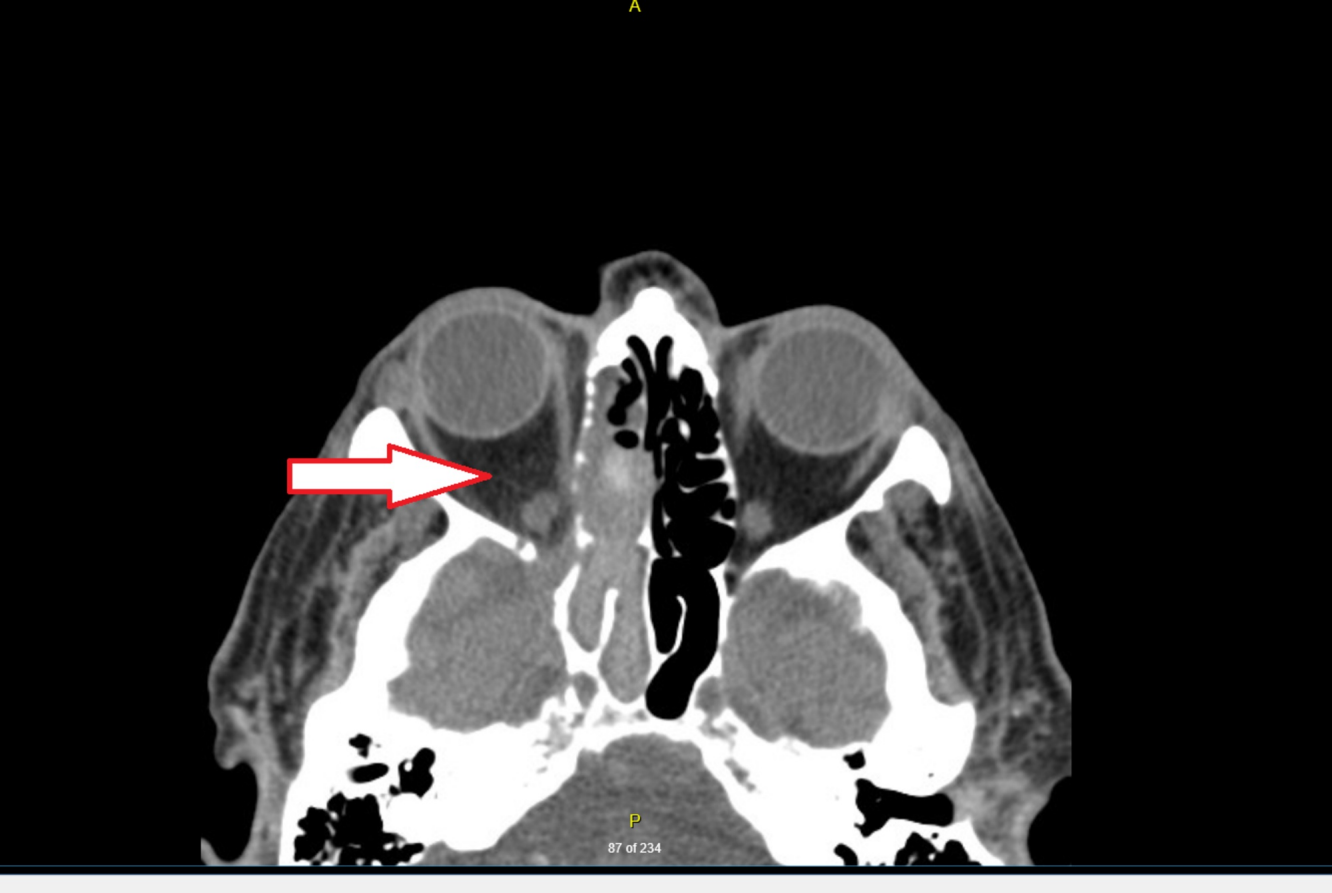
CTA head consistent with invasive fungal sinusitis involving the right paranasal sinuses (arrow). CTA: Computed tomography angiography
